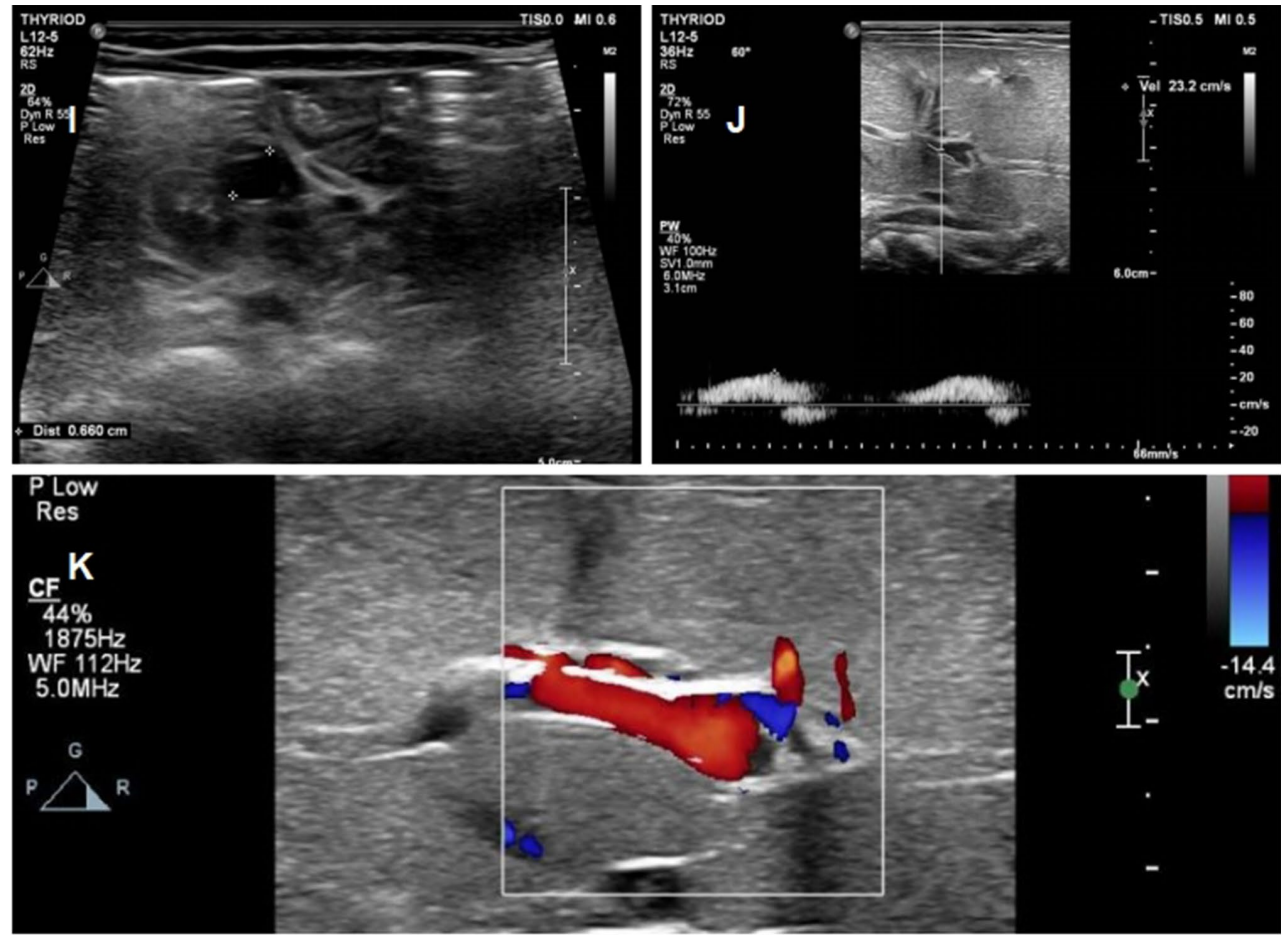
Ultrasound images of the patients on day 26 of life (I) Complete regression of the umbilical vein thrombus at day 26; (J) Complete regression of the portal vein thrombus at day 26; (K) normal blood flow signal of portal vein.
